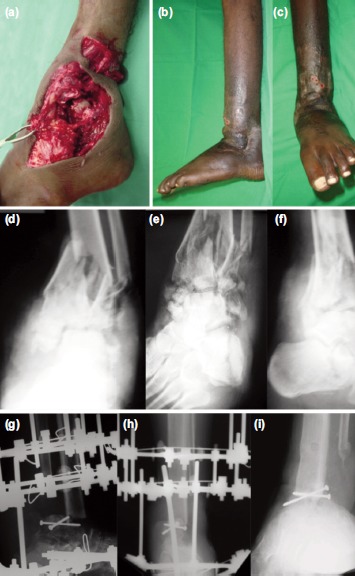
(a) Intra-operative photographs showing comminuted fracture left ankle. (b,c) Photographs after ankle fusion and removal of Ilizarov. (d,e) Pre-operative radiographs anteroposterior, mortis and lateral views showing comminuted fracture left ankle. (f,g) Immediate postoperative radiographs showing ankle arthrodesis with Ilizarov anteroposterior, lateral views. (h,i) Fifteen months post-operative radiographs showing fusion Ilizarov anteroposterior, lateral views.
